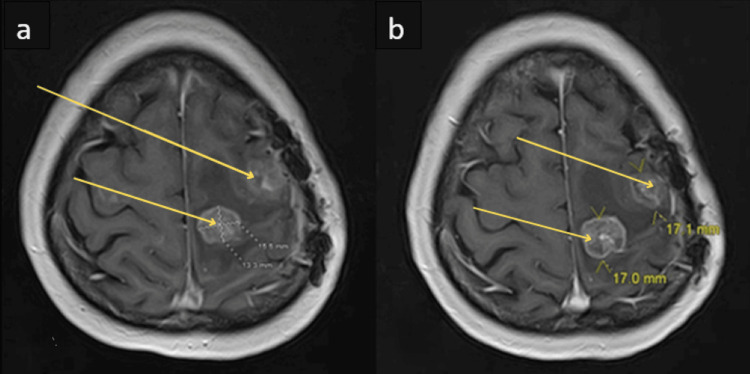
Progressive Enlargement of the Medial Frontal Lesion on Serial Magnetic Resonance Imaging (a) T1-weighted magnetic resonance imaging (MRI), performed approximately 11 months after the initial craniotomy, showing worsening medial edema, with the lesion now measuring 13.3 × 15.5 mm (arrows). (b) Follow-up T1-weighted MRI, performed approximately 13 months post-craniotomy, demonstrating a further increase in vasogenic edema, with the medial lesion enlarged to 17 mm (arrows), despite prior antiparasitic and corticosteroid therapy.
